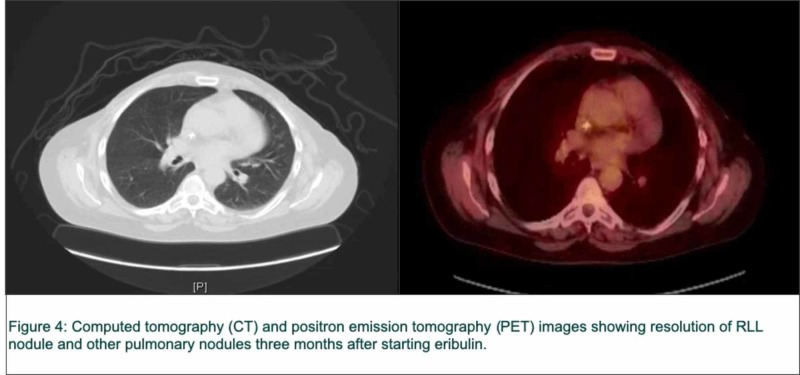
Computed tomography (CT) and positron emission tomography (PET) images RLL: right lower lobe.
